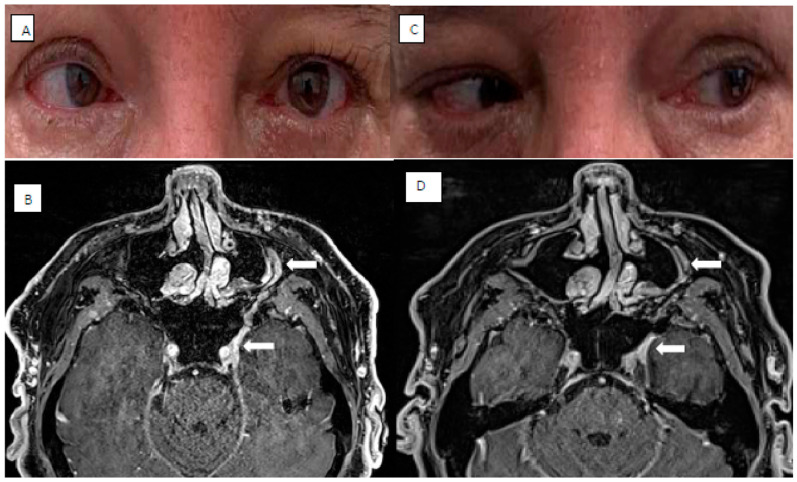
A man presented with a 6-month history of left forehead numbness, diplopia and reduced extraocular left eye movements on a background of resected facial cutaneous squamous cell carcinoma. On examination, there was reduced sensation over the left upper two-thirds of his face and ophthalmoplegia of the 3rd, 4th and 6th cranial nerves with normal optic nerve function (Panel A). A neural-enhanced MRI revealed enhancement of both the frontal branch of the ophthalmic division of the Trigeminal nerve (V1) extending back to the superior orbital fissure and the maxillary division of the Trigeminal nerve (V2) with atrophy of the superior oblique muscle consistent with perineural spread (Arrows; Panel B). The patient commenced Programmed Death Receptor-1 (PD-1) inhibitor with Cemiplimab. Following 3 months of treatment, his ophthalmoplegia improved on lateral gaze (Panel C) with regression of the neural involvement on MRI showing reduced perineural thickening and contrast enhancement (Arrows; Panel D).
